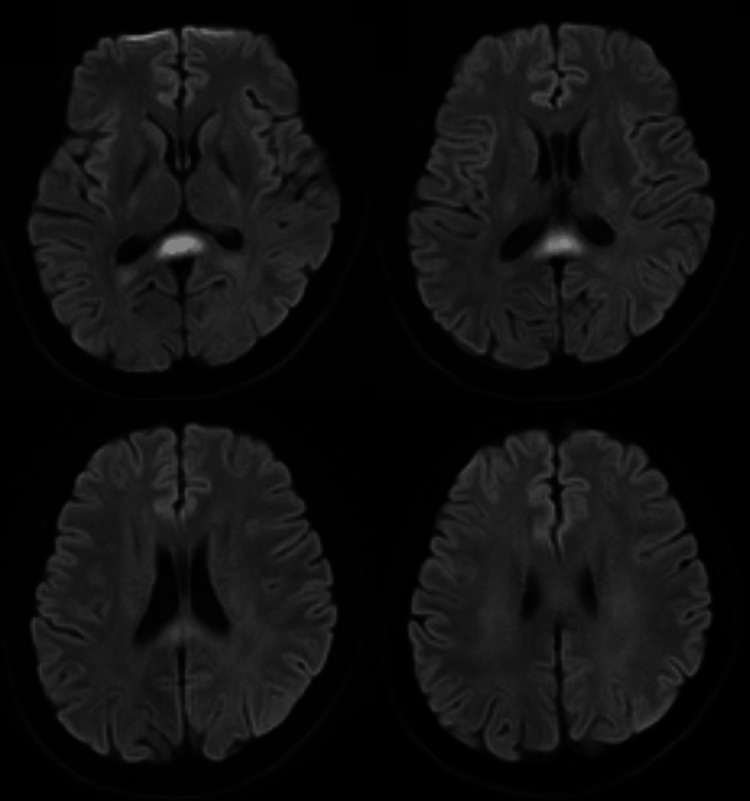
Follow-up MRI performed five days after the initial scan showing near-complete resolution of the previously noted DWI hyperintensities. MRI: magnetic resonance imaging; DWI: diffusion-weighted imaging
